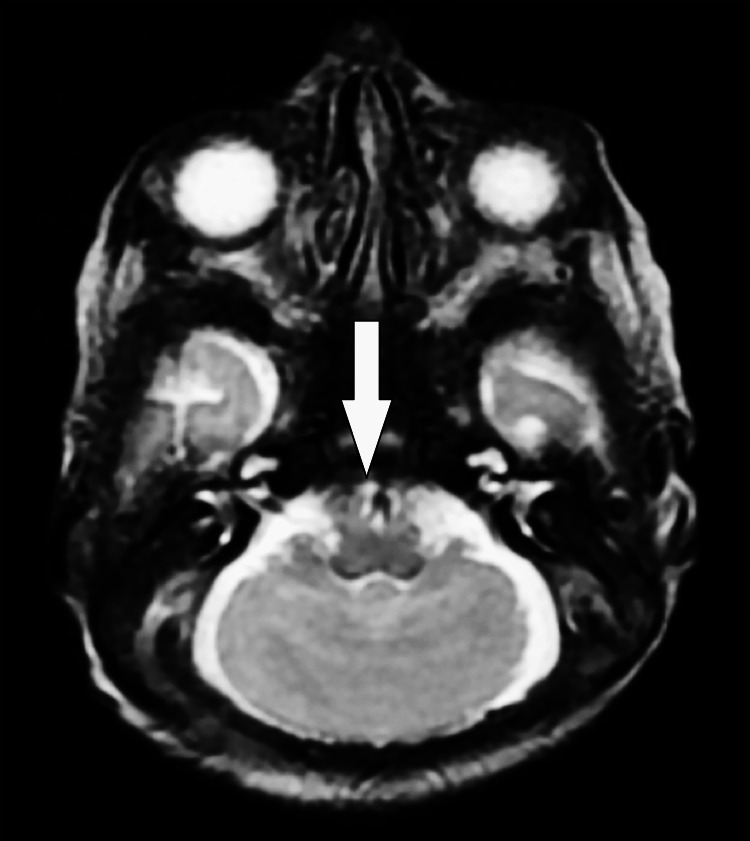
Axial view MRI T2WI axial view shows fused cerebellar hemispheres across the midline with the absence of vermis highlighted by the 'Donald Duck' sign with dilated lateral and third ventricle secondary to aqueductal stenosis T2WI: T2 weighted image
